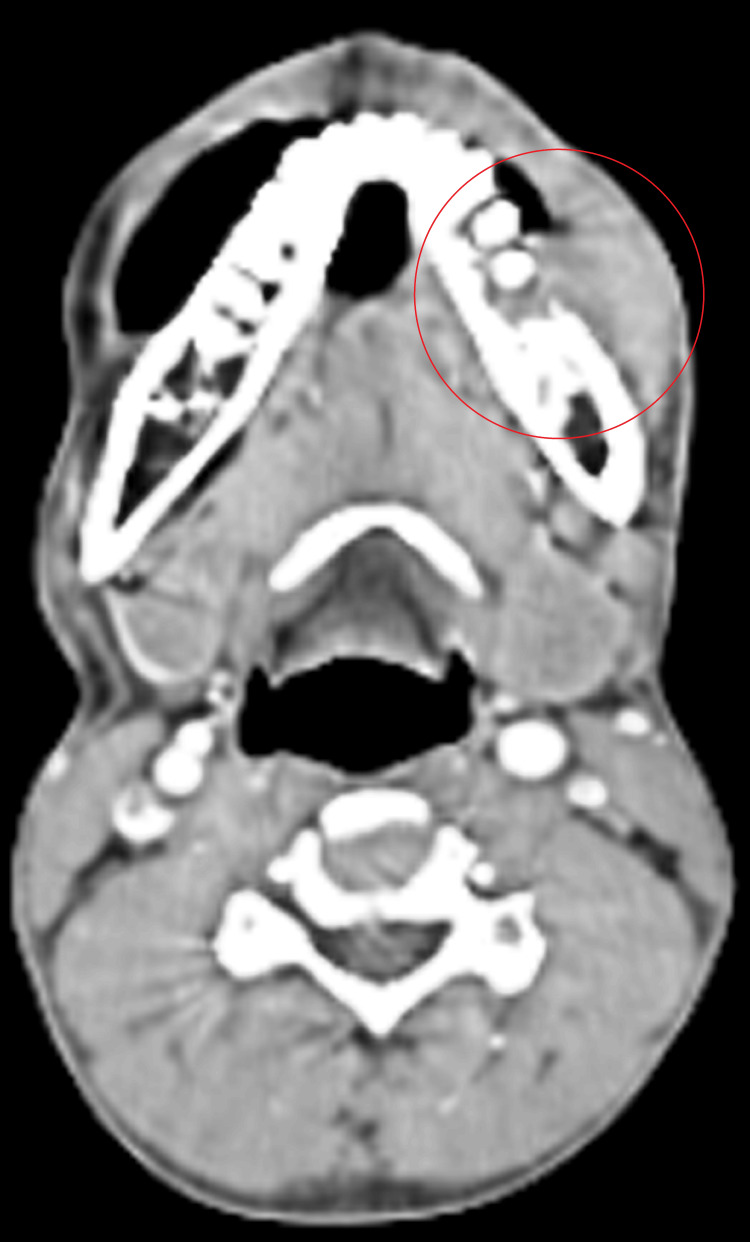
Computed tomography revealing a lesion in the left lower gingiva buccal sulcus.
